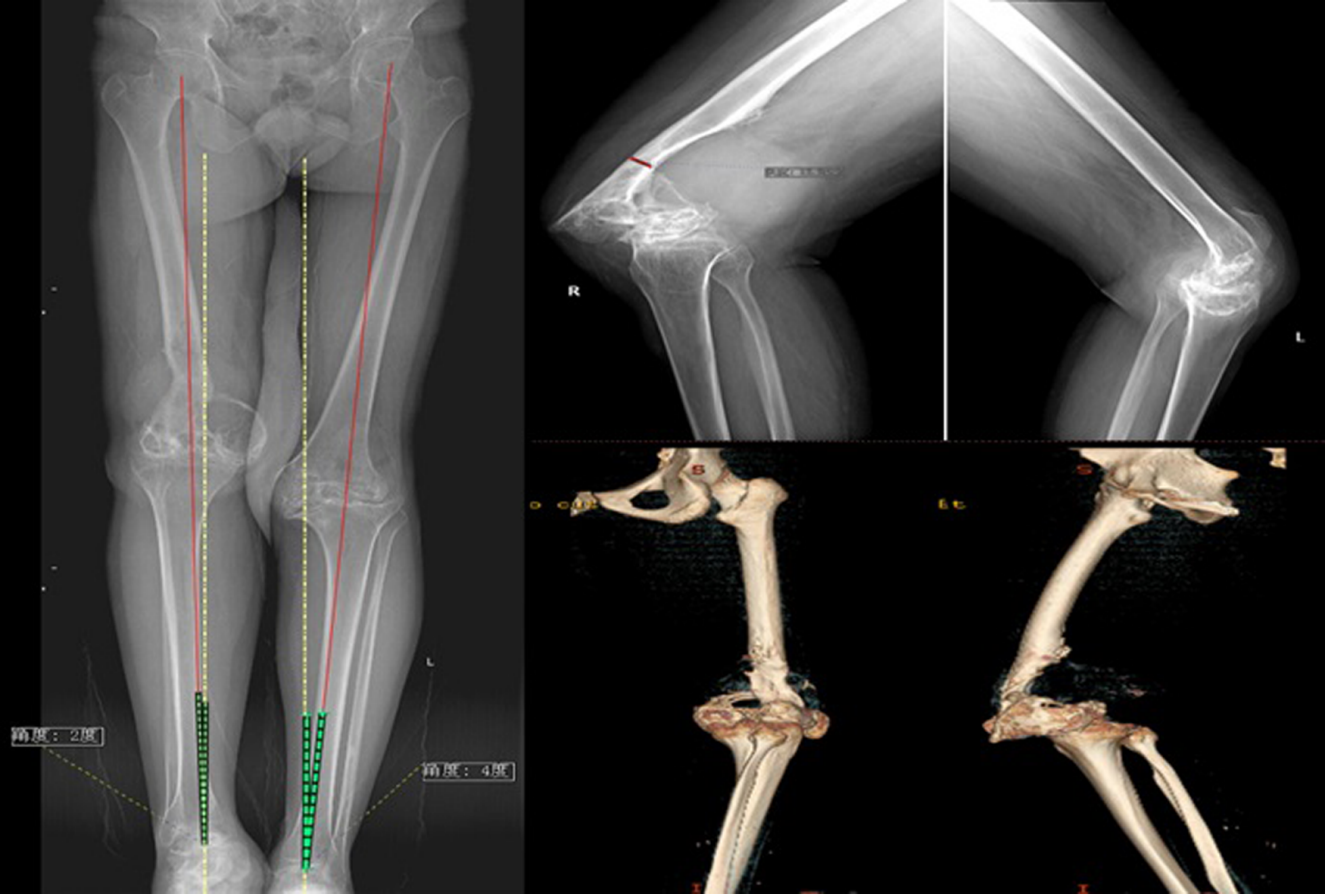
Preoperative roentgenograms and CT reconstruction of hemophilic arthropathy of the knee.
